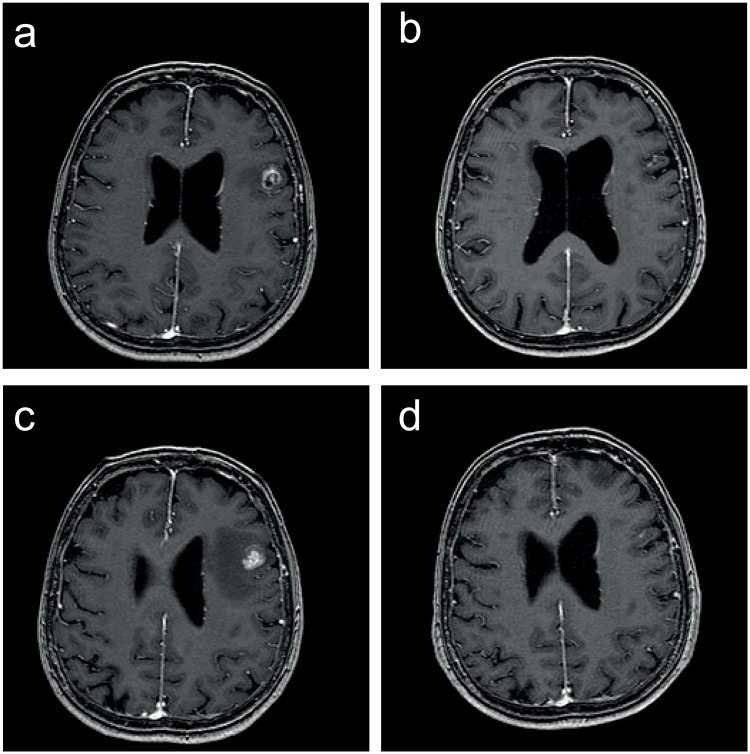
MRI images of a recurrent metastasis. a) before and b) after initial treatment, c) before and d) after Re-SRS.
